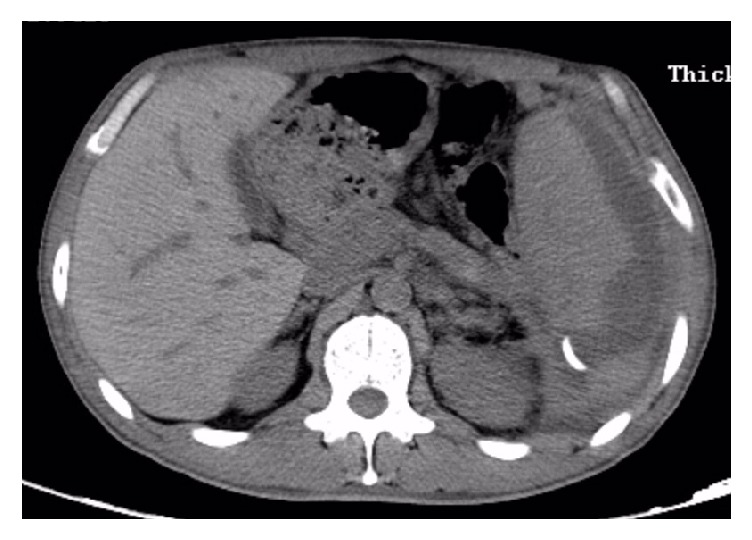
About 2 weeks after splenic artery embolization and ultrasound-guided percutaneous drainage of pancreatic pseudocyst, CT showing the disappearance of pancreatic pseudocyst, multiple areas of infarction on the spleen, and the significant reduction of splenic subcapsular hematoma.
